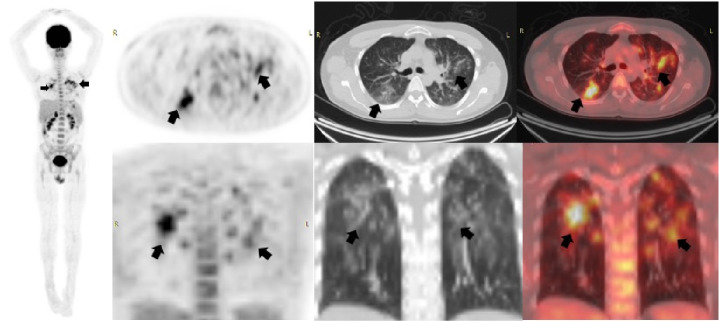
PET/CT F18-FDG en la cual se observaron múltiples opacidades nodulares y seudonodulares de varios tamaños, con incremento glucolítico y patrón de vidrio esmerilado, dispersas aleatoriamente en ambos parénquimas pulmonares con patrón multisegmentario dominante en los lóbulos superiores, incremento glucolítico dominante en el segmento anterior del lóbulo superior izquierdo y SUVmáx de 5,6, múltiples nódulos de menos de un centímetro (promedio de 5 mm), coalescentes, y opacidades en parches con patrón de vidrio esmerilado, mal definidas y bilaterales (flechas negras).
